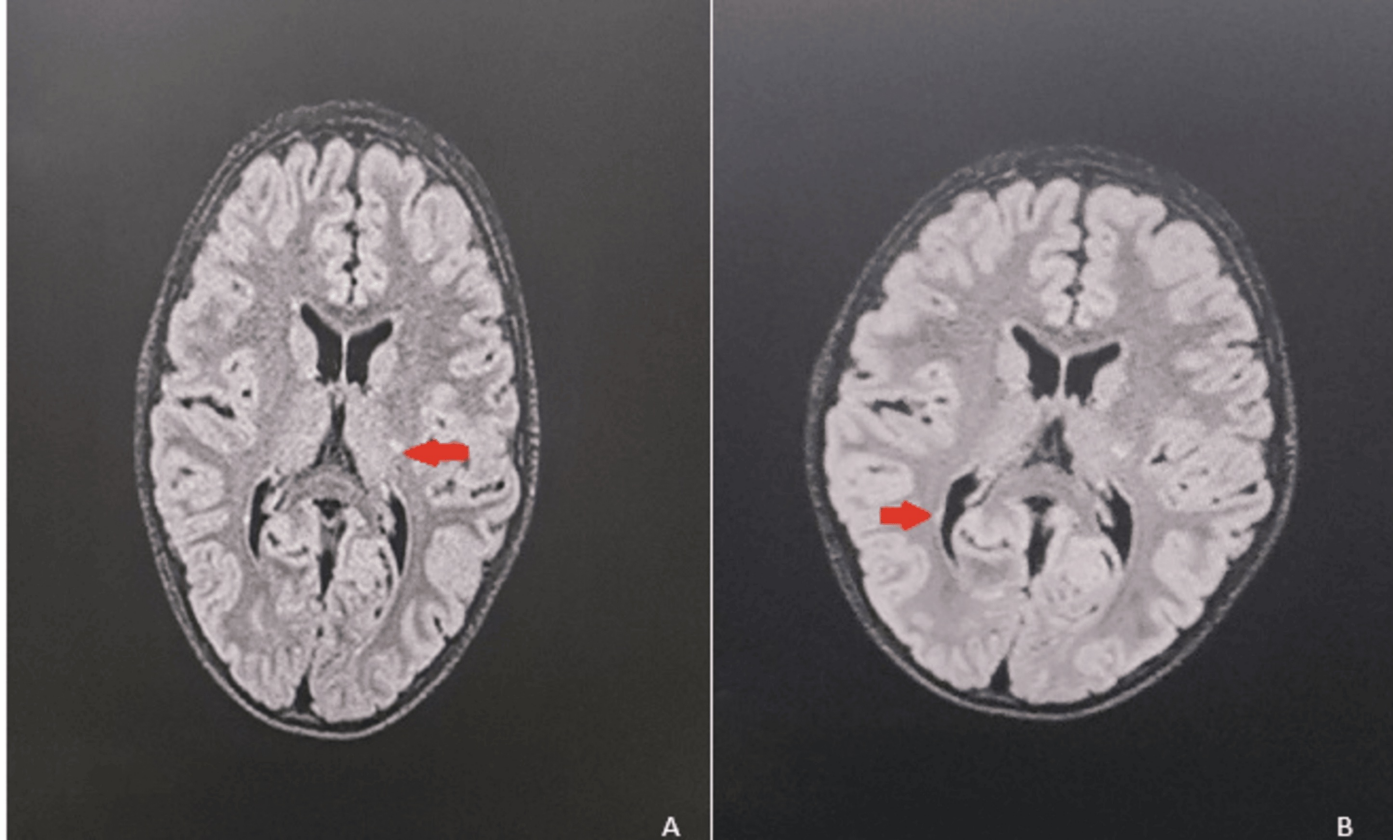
Axial T2/FLAIR brain MRI showing periventricular demyelinating lesions. (A) Left periventricular white matter lesion in the region of the posterior internal capsule adjacent to the left lateral ventricle (red arrow). (B) Additional periventricular demyelinating lesion (red arrow) with characteristic features of pediatric multiple sclerosis, including periventricular distribution and T2 hyperintensity. MRI: magnetic resonance imaging; FLAIR: fluid-attenuated inversion recovery
